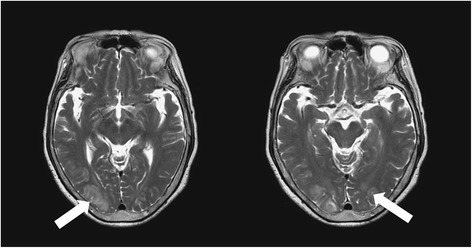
Brain MRI of our patent on the 4^th^ day. Brain MRI T2-weighted hyper-intense signal abnormalities in the occipital lobes bilaterally
